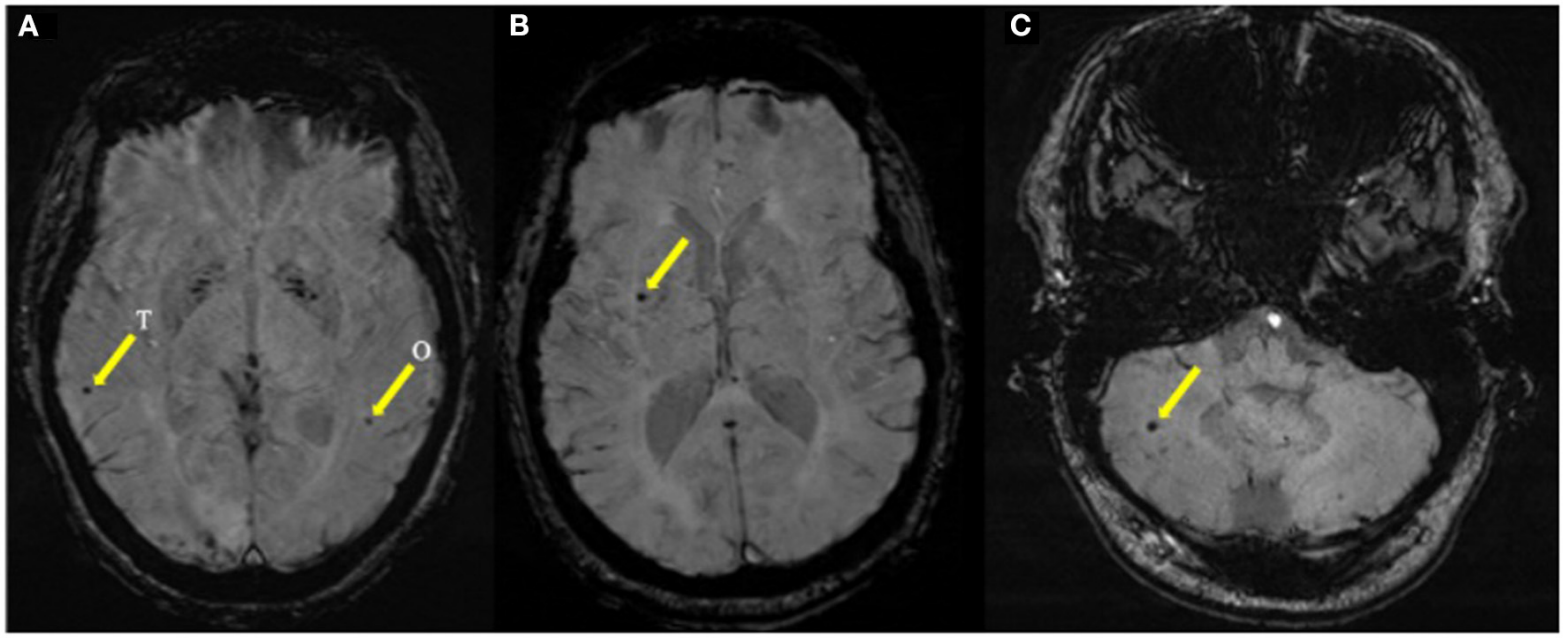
Representative MRI images of CMB in ischemic stroke patients. (A) Representative SWI sequence in a 72 years-old black female demonstrating two lobar CMB in left occipital lobe (O) and right temporal lobe (T). (B) Representative SWI sequence in a 66 years-old black male demonstrating a deep CMB in the basal ganglia. (C) Representative SWI sequence in a 56 years-old white male demonstrating an infratentorial CMB in the cerebellum.
